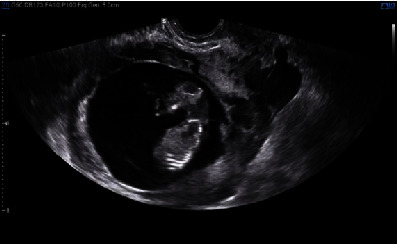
Ultrasound imaging with radiology report noting thickening of the endometrium at the fundus. The gestational sac appears at the lower segment with bulging towards the bladder. On the transvaginal area, there is significant vascularity surrounding the gestational sac and there appears to be very little myometrium.
